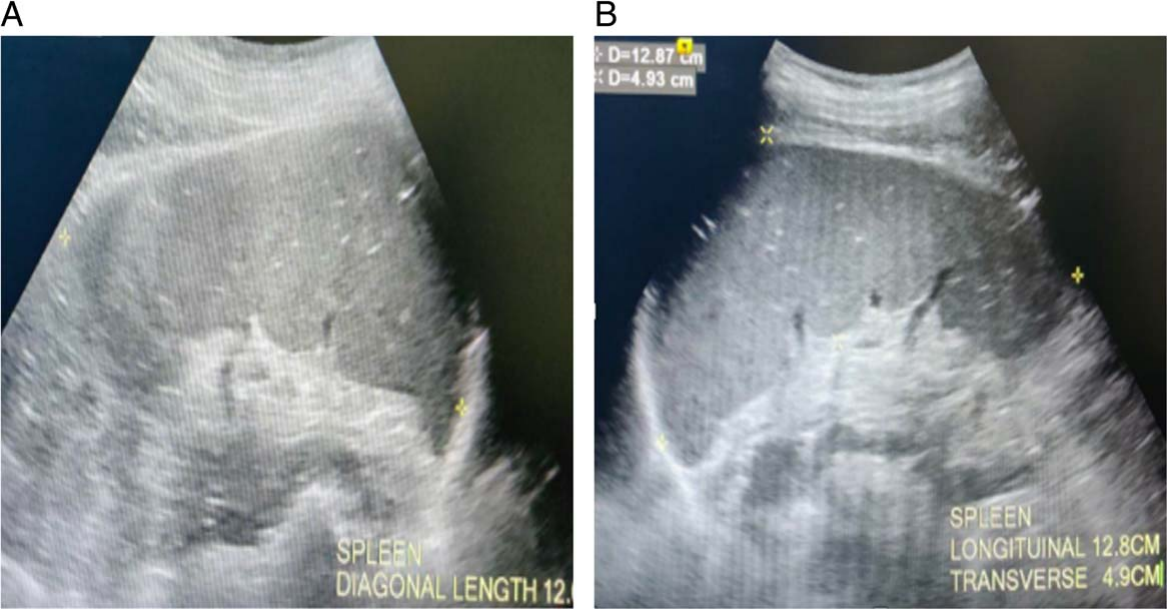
A: Greyscale ultrasound image showing the measurement of the diagonal dimension of the spleen. B: Greyscale ultrasound image showing the measurement of the longitudinal and transverse dimensions of the spleen.
